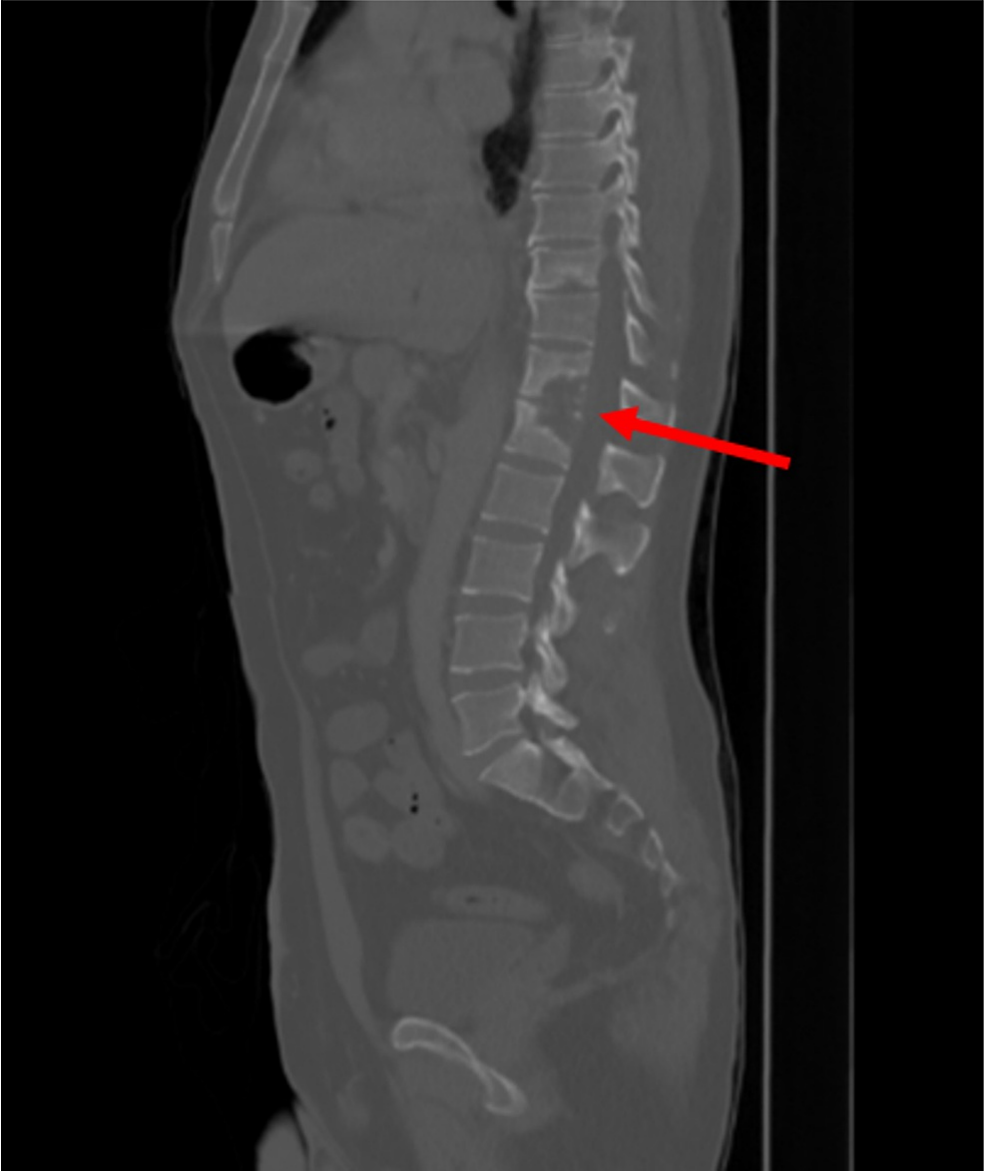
CT scan demonstrating destruction of T12 - L1 vertebral bodies (red arrow)
